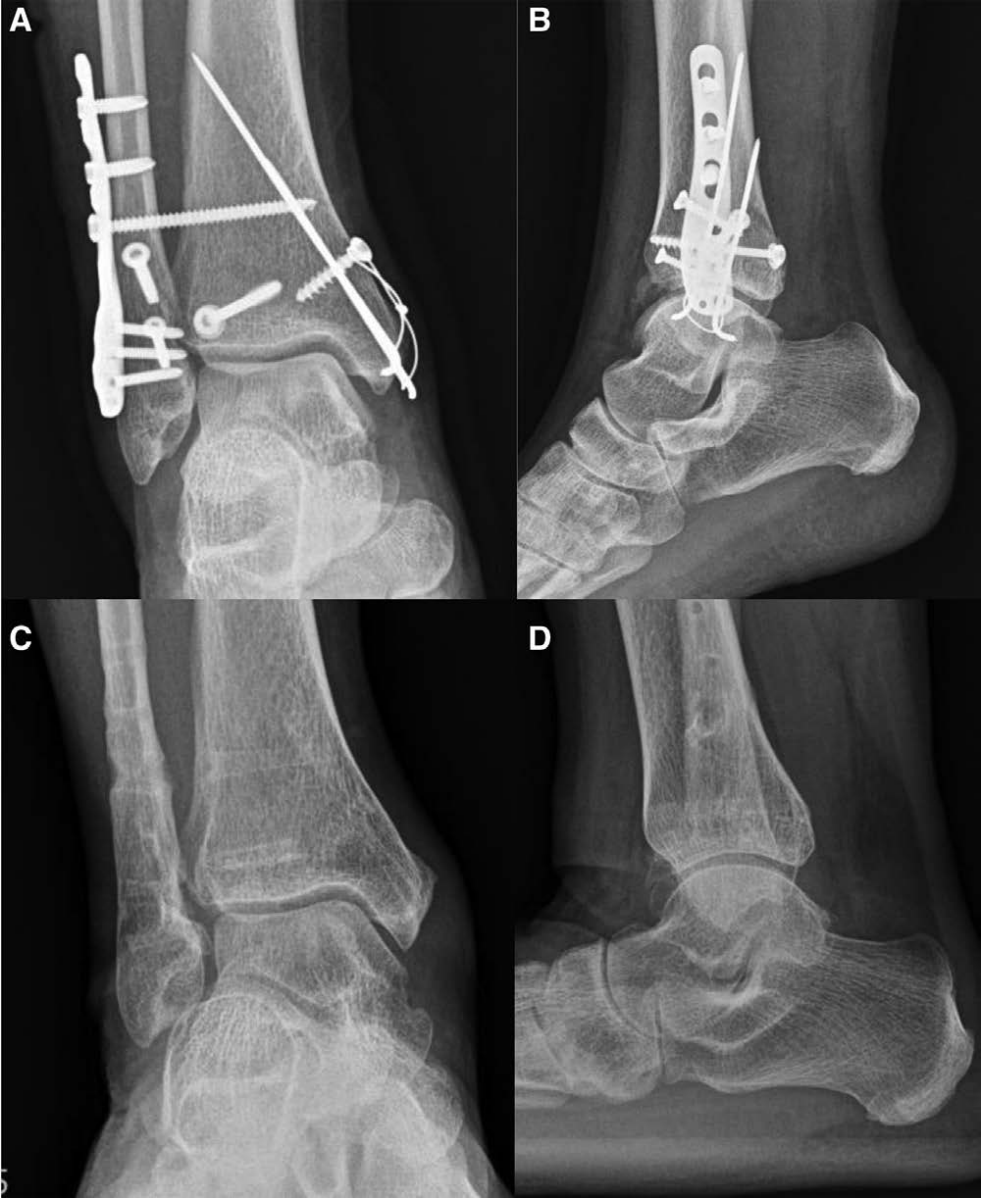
Postoperative image of the patient presented in Figure 1. Immediate postoperative (A) anteroposterior and (B) lateral ankle plain radiographs. Postoperative 1-year (C) anteroposterior and (D) lateral ankle plain radiographs. The articular surfaces are well preserved and there is no evidence of post-traumatic arthritis.
